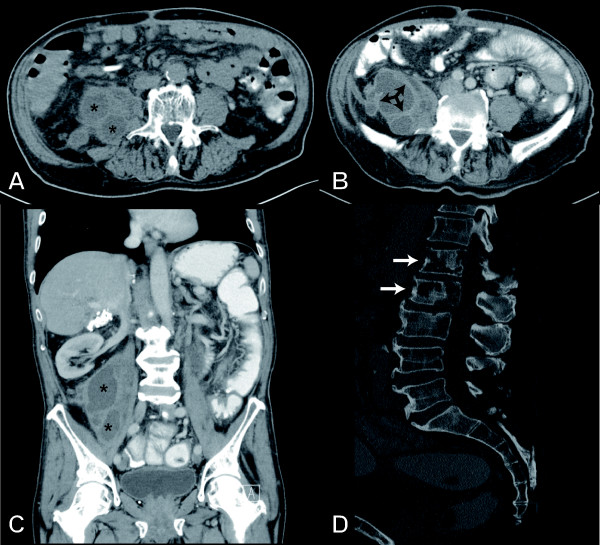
Computed tomography scans of the abdomen and pelvis. Non-enhanced (A) and contrast-enhanced (B) axial images show a multilobulated cystic mass in the right retroperitoneum, originating from the psoas muscle. Besides of the cystic components with fluid-like density (*) thickened septa with mild contrast enhancement (arrow) are seen. The coronal image (C) shows the extent of the mass along the psoas muscle. (D) The lumbar spine presents lytic lesions of the first and second lumbar vertebra (arrows) with partial cortical destruction.
